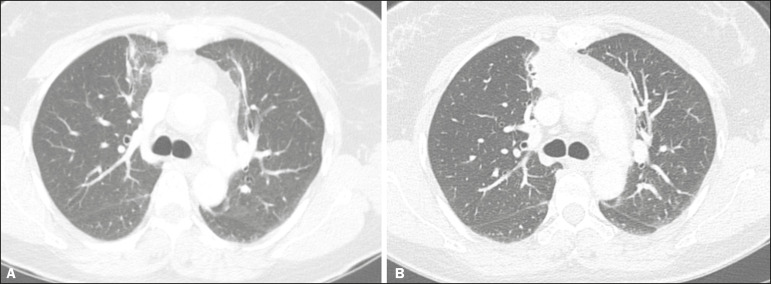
CT scan of a patient with a history of thymoma (A), showing ground-glass opacities in the paramediastinal regions, especially on the right. Follow-up CT scan, acquired at 8 months after radiotherapy (B), showing a predominance of retractile opacities and volume reduction, suggesting that the radiation-induced lesion had evolved to the fibrotic phase.
